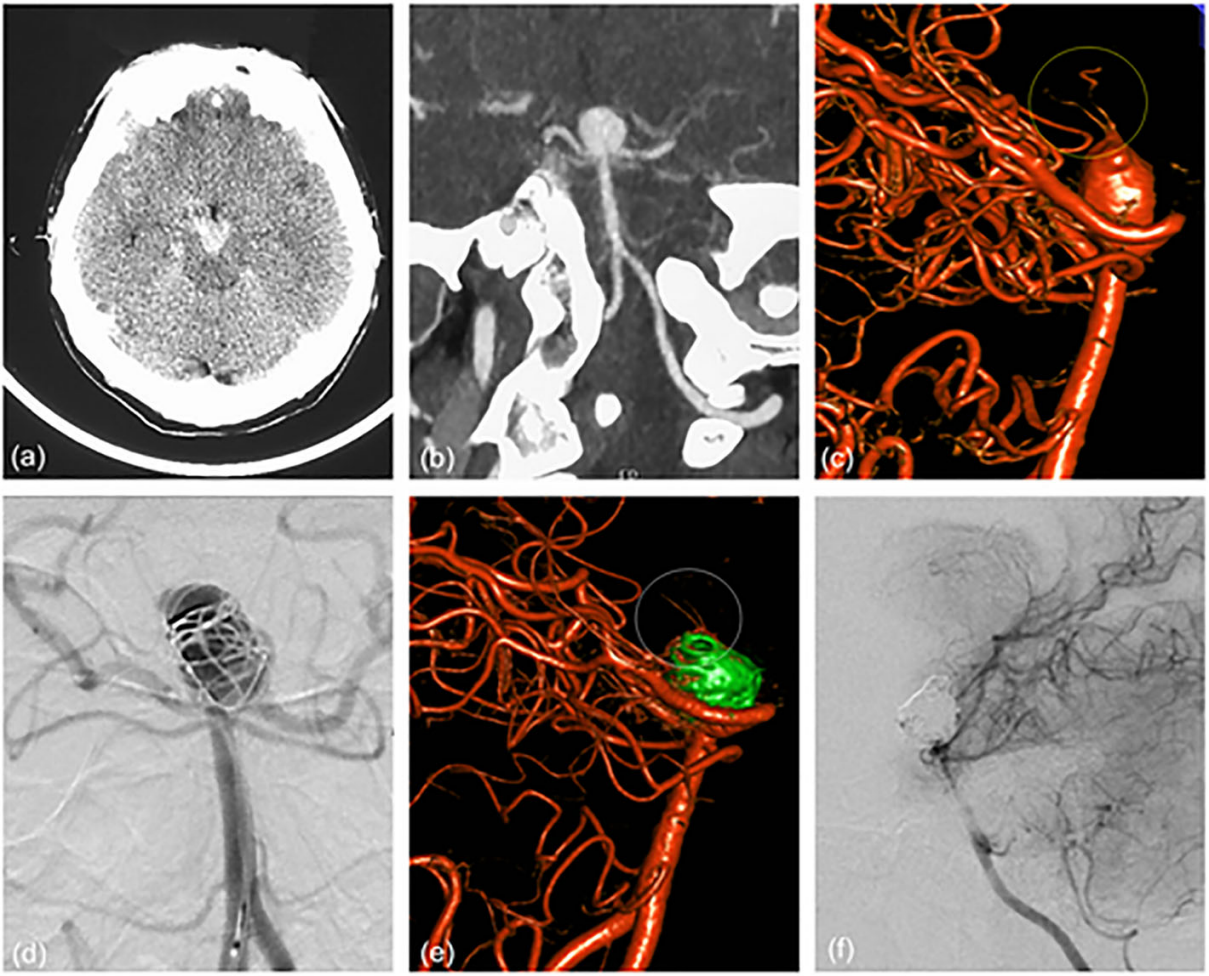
a, b Patient 2 presented with acute SAH and the initial CTA showed a huge aneurysm at the tip of the basilar artery. c The 3D rotational angiogram showed many perforating arteries on the dome of the aneurysm (yellow circle). d We used different diameters coils to embolize the main part of the aneurysm body, leaving a certain space at the dome of the aneurysm. e Postoperative angiography showed satisfactory embolization of the aneurysm and perfect preservation of perforating arteries on the dome of the aneurysm (white circle). f Follow-up angiography after 4 months showed no aneurysmal recurrence and perfect preservation of the lenticulostriate artery
